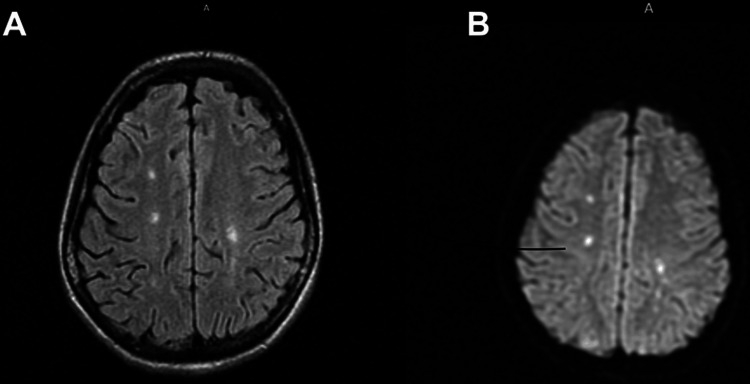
Brain MRI showing white matter hyperintensities on T2-FLAIR (1A) and DWI (1B), compatible with several acute stroke lesions.
